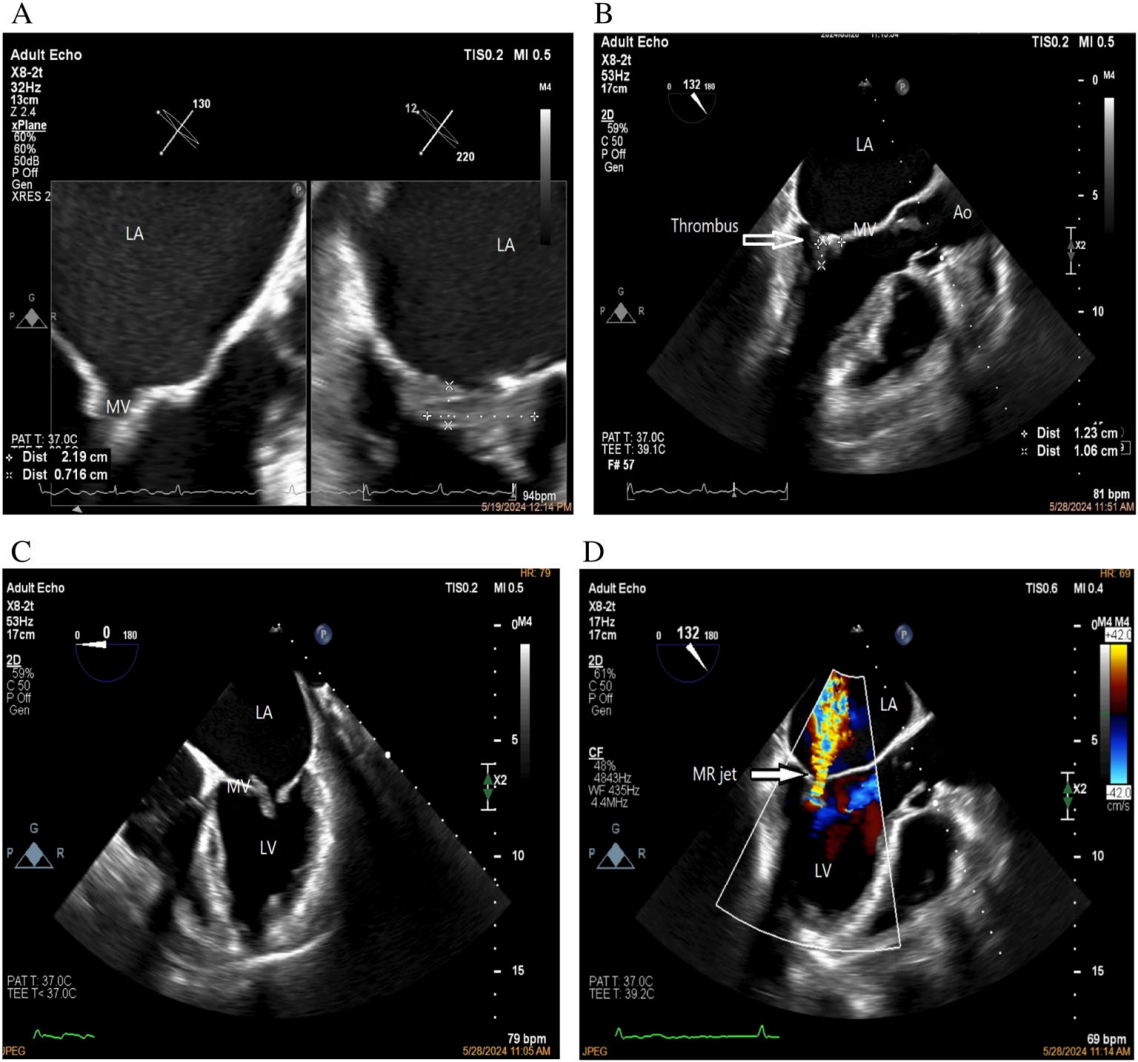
(A) Two‐dimensional transesophageal echocardiography (TEE) showing a hypoechoic mass around the leaflet tips, suggestive of thrombus or vegetation (size = 2.19 × 0.71 cm). (B) Two‐dimensional transesophageal echocardiography (TEE). Control echocardiography after 1 week of admission revealed a significant decrease in the size of the mass (size = 1.23 × 1.06 cm). (C) Two‐dimensional transesophageal echocardiography (TEE), esophageal view, showing mitral valve opening in mid‐diastole. A hypoechoic mass was observed on the anterior mitral valve leaflet (AMVL), and opening of the MV was restricted. (D) Two‐dimensional transesophageal echocardiography (TEE). Colored doppler shows a moderate regurgitant flow of mitral valve (MV).
